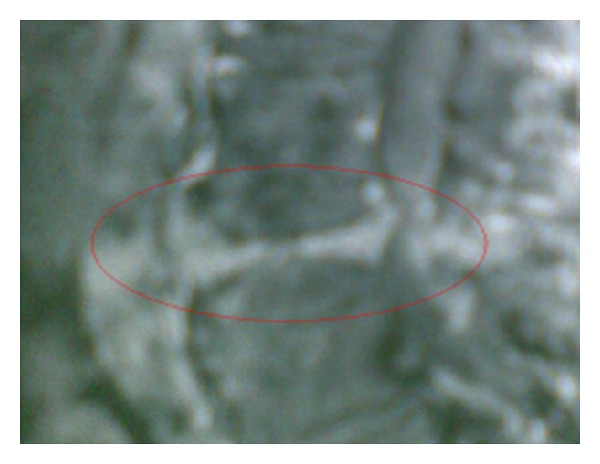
MRI: T1 low sign: L3 compaction.
